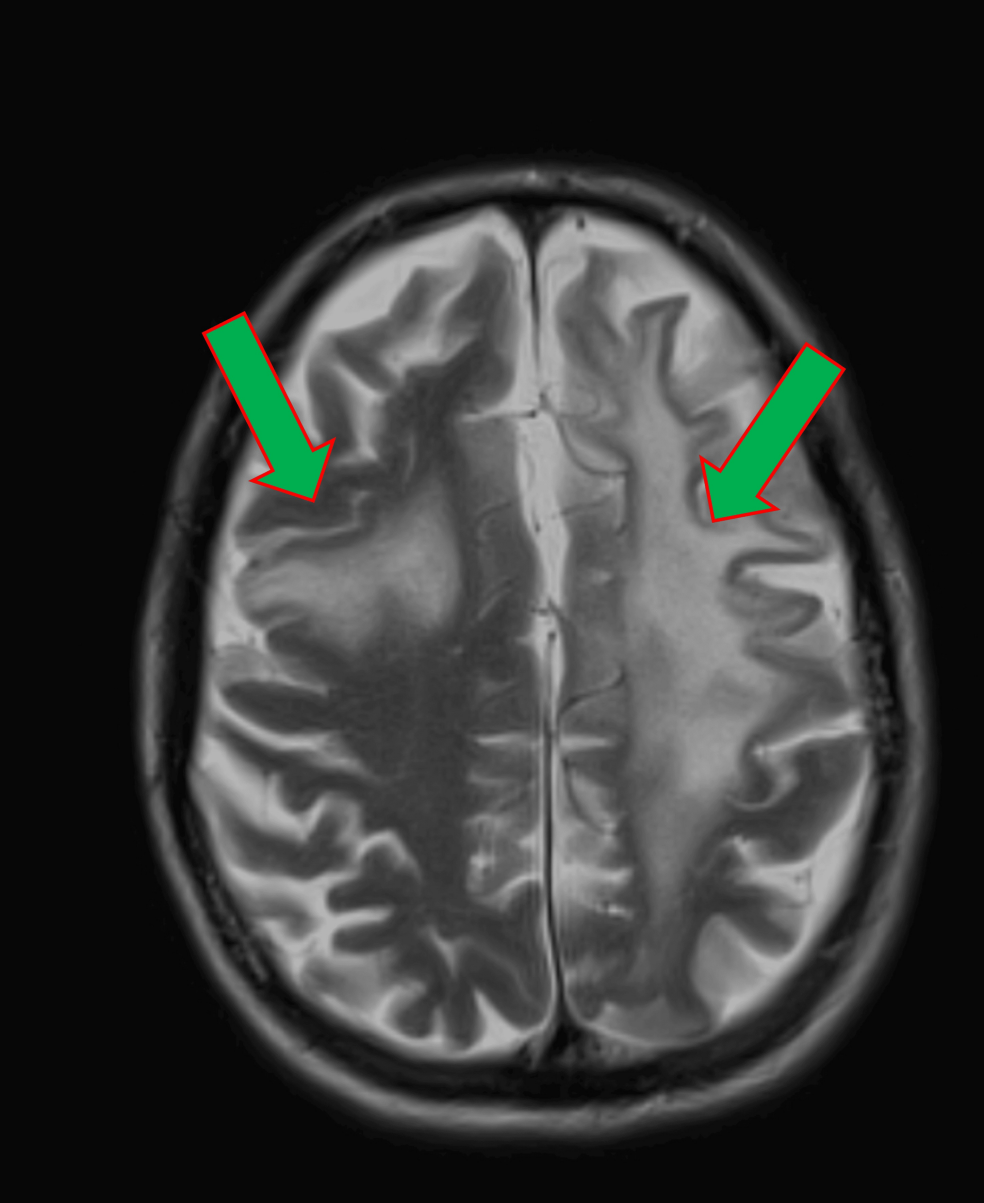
MRI T2-weighted image showing approximately a 7.4 × 3.3 cm-sized left frontal lobe white matter lesion and a smaller but similar lesion (about 1.5 cm) in the right frontal lobe.
